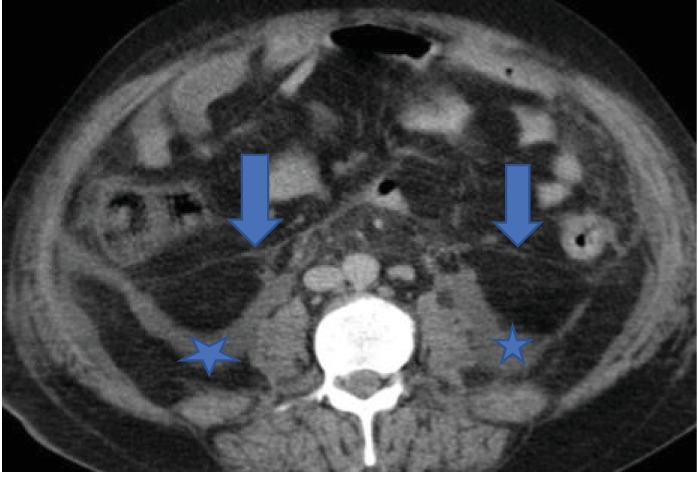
Axial CT image of the abdomen and pelvis at level of L2 showing fluid (asterisk) in bilateral posterior pararenal spaces and thickening of anterior pararenal fascia (arrows).
